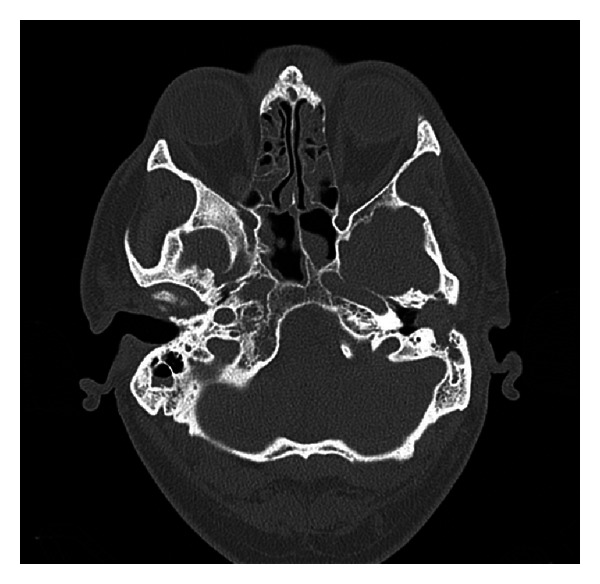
CT petrous bones axial image showing left middle ear soft-tissue mass.
